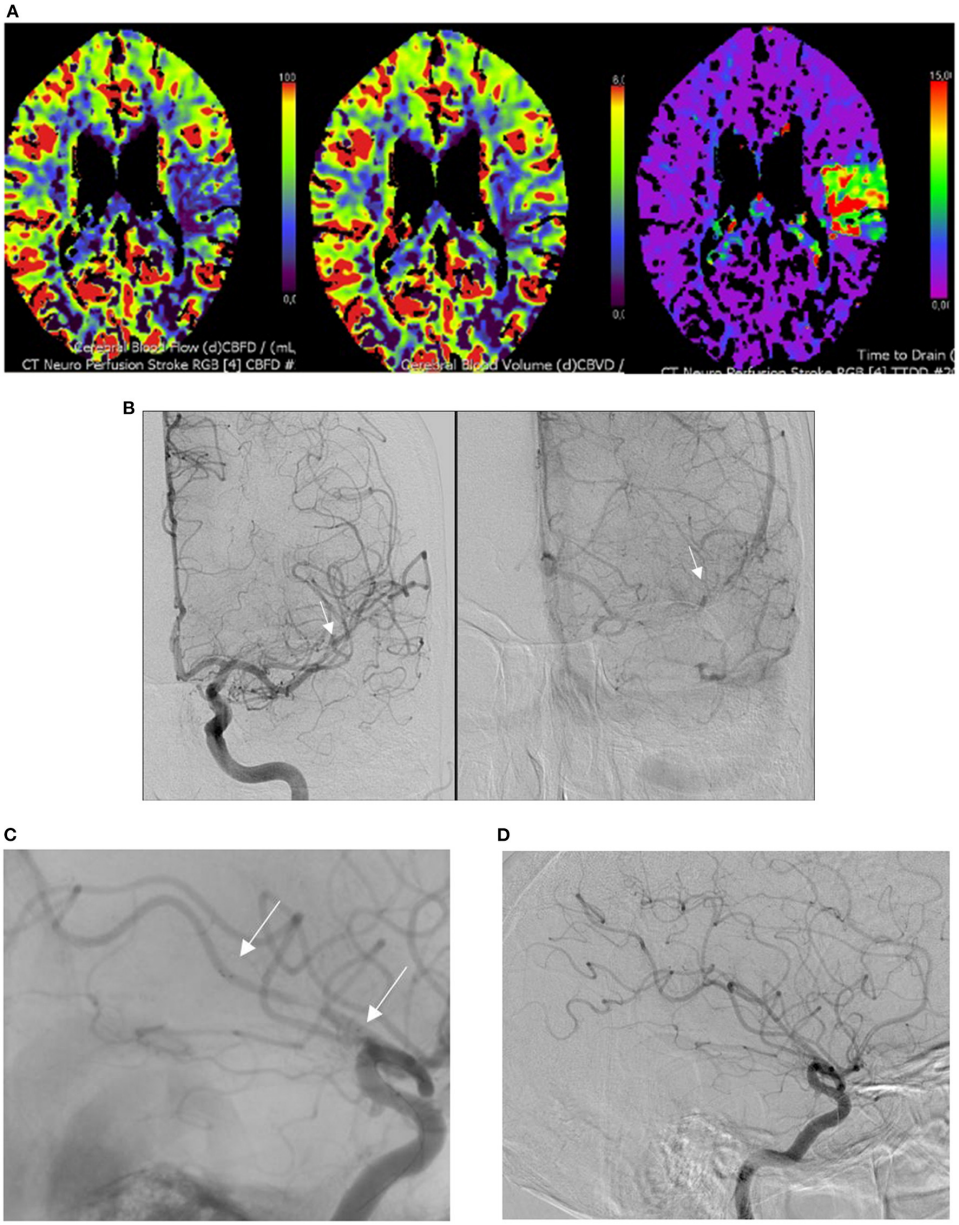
M2 occlusion treated with the CatchView Mini device. (A) Perfusion computed tomography demonstrating a decrease in blood flow with normal volume and an increase in mean transit time in the territory of the left M2 segment of the middle cerebral artery. (B) Visualization of the M2 occlusion on angiography (anteroposterior view, white arrows). (C) Visualization of the distal and proximal ends of the opened CatchView Mini stentriever device inside of the clot on angiography (white arrows). (D) Final angiography post-procedure demonstrating complete recanalization of the occluded vessel.
